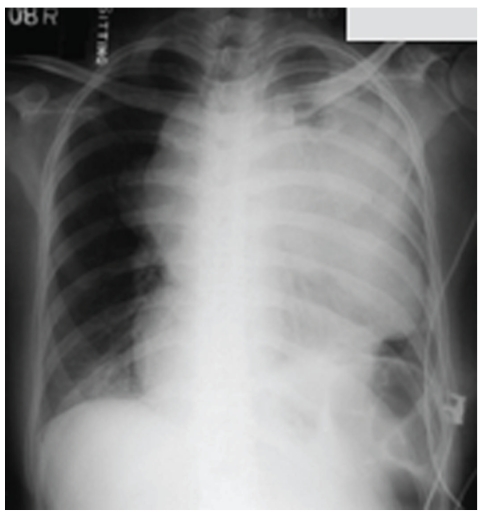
Chest X-ray was taken with abdominal shield. There was a huge mass occupying the left chest; trachea is deviated to the right. The mass caused loss of volume in left lung and elevated left hemidiaphragm.
